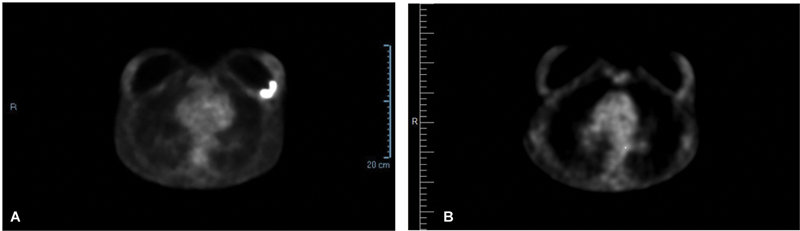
PET-CT scan images taken before and after neoadjuvant chemotherapy and targeted therapy. (
